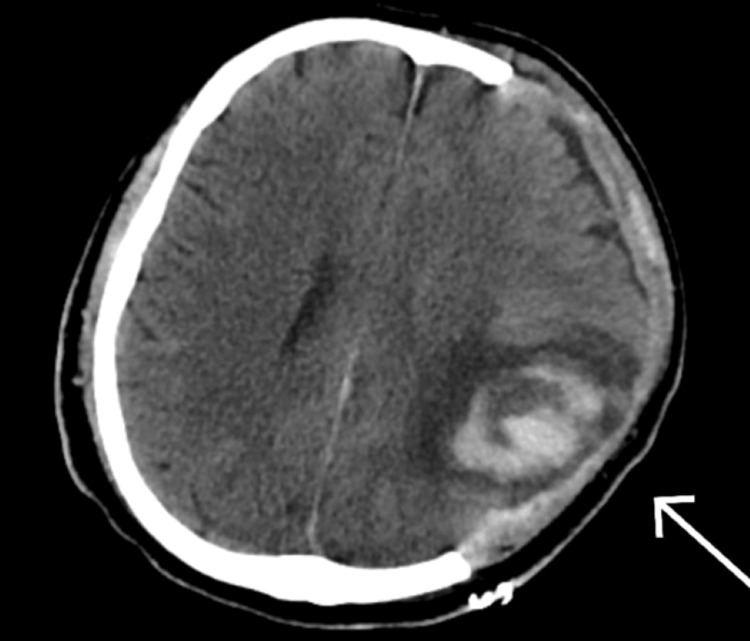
CT brain post-operatively, with the arrow representing post-craniectomy changes, as mentioned in the text.
